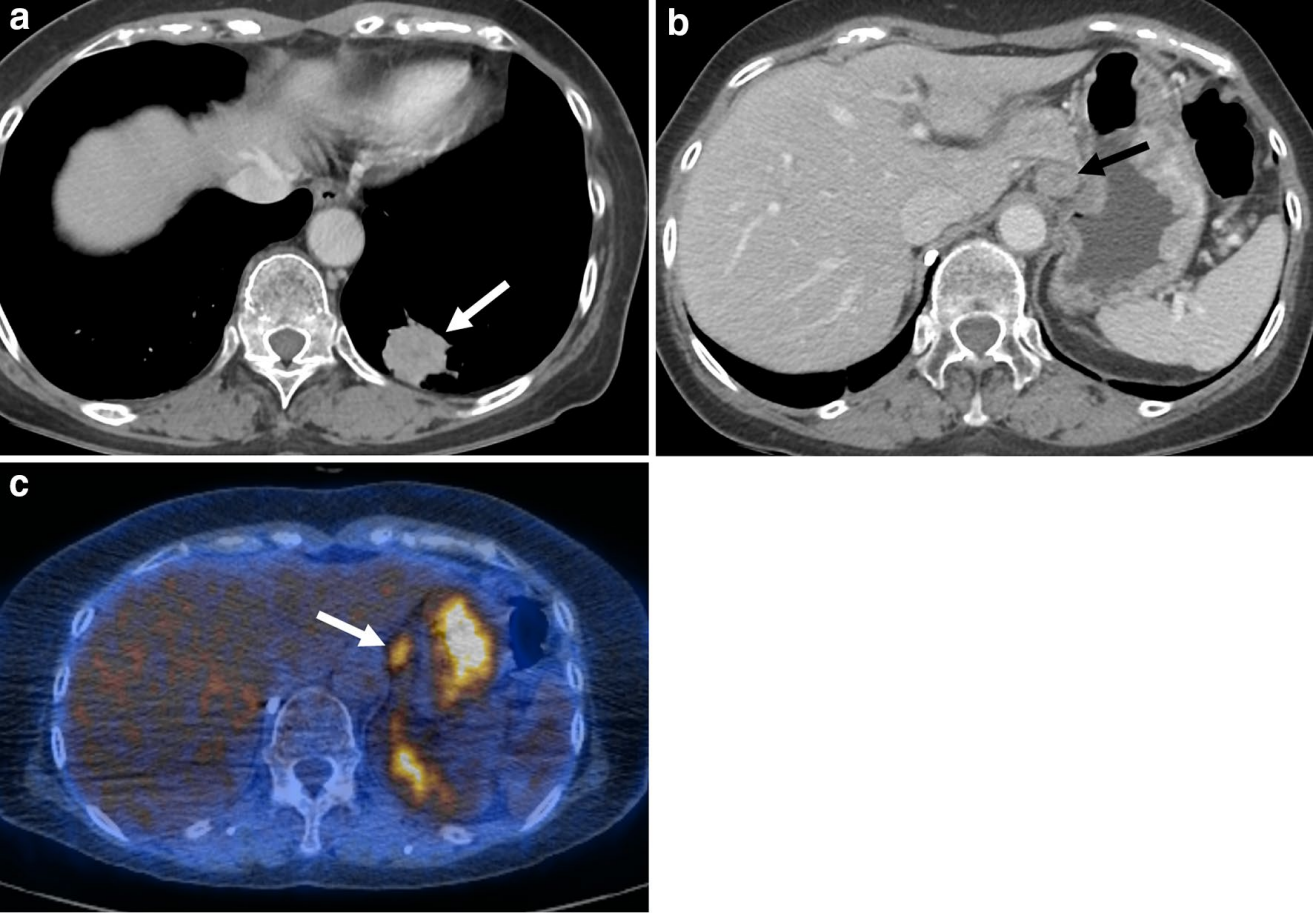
A 55-year-old woman with *ROS1*-rearranged lung adenocarcinoma with distant (abdominal) lymph node metastases. (a, b) Chest CT images demonstrate a peripheral solid mass (arrow) in the left lower lobe, which was shown to be adenocarcinoma from the percutaneous core needle biopsy. Note the enlarged left gastric lymph node (arrow in b). (c) Fused PET/CT image demonstrates FDG-avid left gastric lymph node (arrow), which was revealed to be metastasis from the endobronchial ultrasound-guided needle biopsy.
